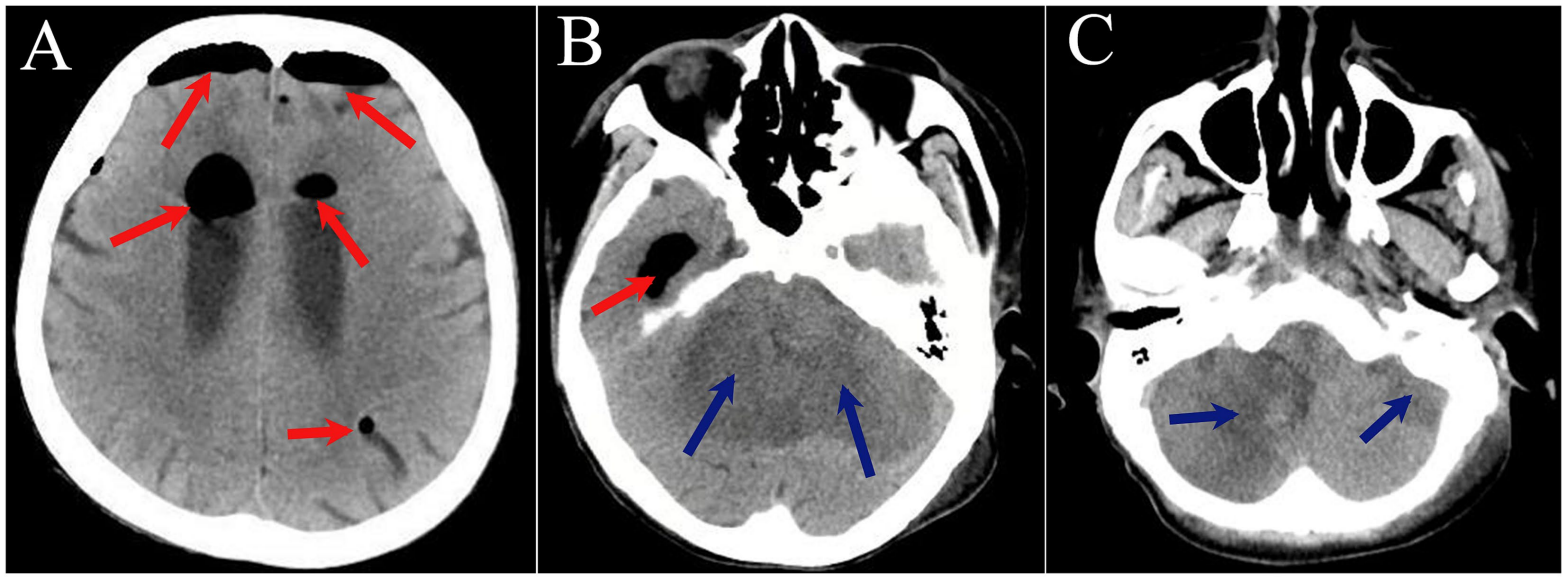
(A–C) CT of the head. Red arrows indicate pneumocephalus involving the intracranial space and ventricular system; green arrows indicate hypodense lesions in bilateral cerebellar hemispheres.
